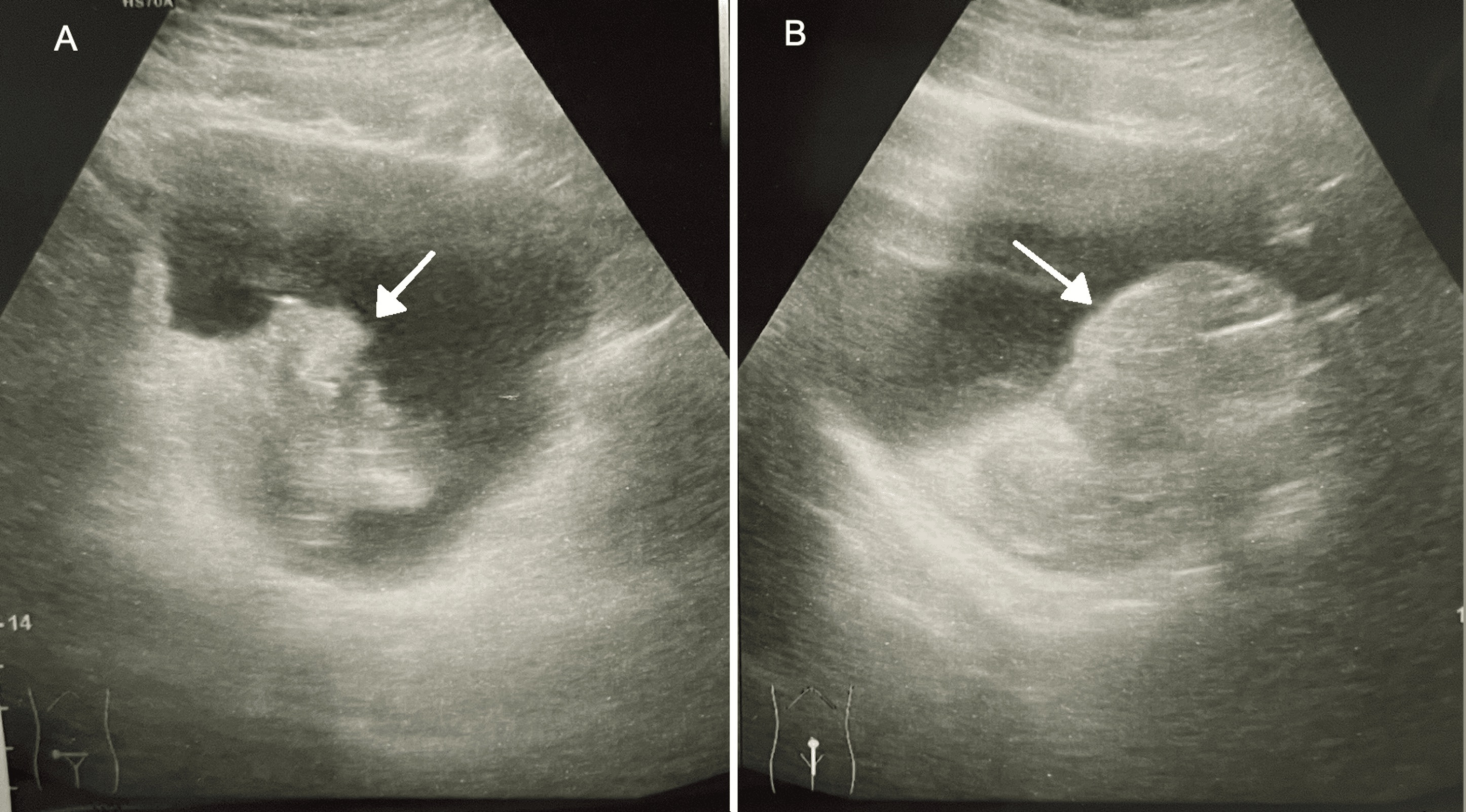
Ultrasound images of the bladder. The left panel (A) shows a transverse view, while the right panel (B) shows a longitudinal (sagittal) view. In both images, a white arrow indicates an echogenic lesion located at the dependent portion of the bladder base, which may represent either a blood clot or a tumour.
